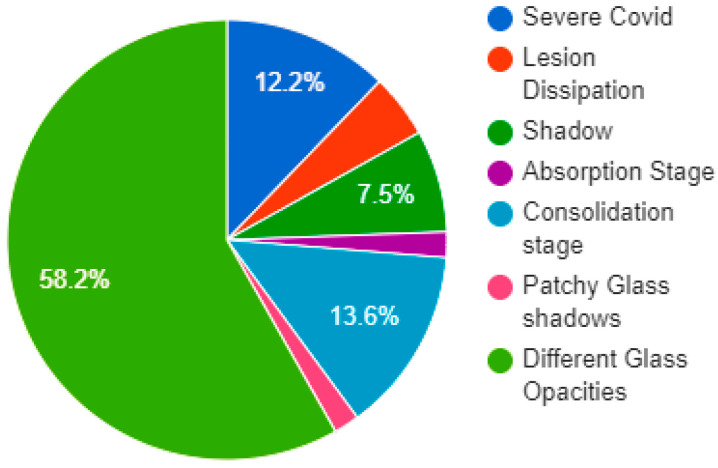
Chart of distribution of the proportion of CT manifestations of COVID-19.
